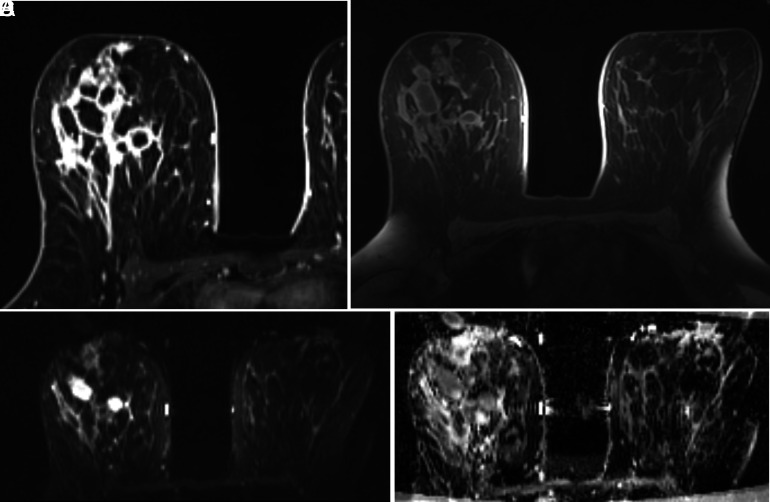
Idiopathic granulomatous mastitis (IGM) in a 24-year-old patient with a persistent history of IGM on the right breast for the past 3.2 years. A thorough evaluation was not yielding for any etiologic agent. The lesions on magnetic resonance imaging (MRI) show extensive segmental involvement with diffuse contrast enhancement on (A) flow dynamic and (B) post-contrast subtraction images. The mass shows restricted diffusion on (C), axially acquired image from diffusion-weighted MRI (*b *= 800 s/mm^2^) and a low apparent diffusion coefficient on (D). The patient underwent 5 courses of intralesional steroid injection therapy and no relapse was noted on 3 years of follow-up.
